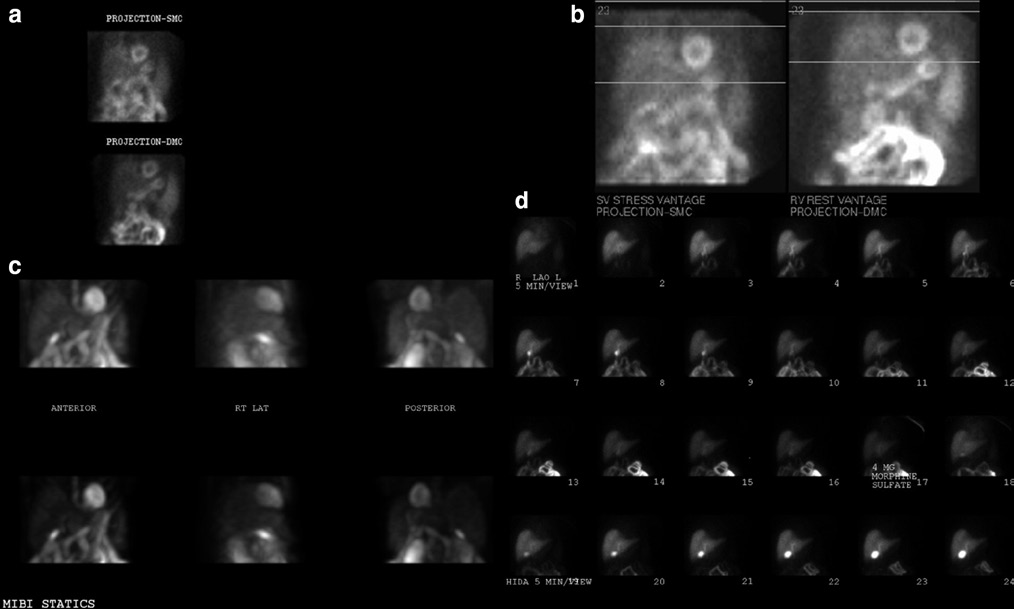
(a) Faintly visualized GB on the stress/rest MIBI data. (b) Enlarged S/R data with different intensity, not convincing enough. (c) Ant., Rt. Lat. and Post. Frames of the 3D reconstructed Stress data, clearly shows the GB. The 3D data in Cine Mode, gives an unequivocal GB visualization in its proper location. (d) HBS shows what appears to be GB at frames 5–9; the Cine Mode reveals passage of the radiotracer through the “C” loop of the duodenum. Post MS04 at frame #17 and a booster dose, the GB appears, in the same location as the 3D data. 3D, three-dimensional; GB, gall bladder; HBS, hepatobiliary scintigraphy; MIBI, methoxy isobutyl isonitrile.
